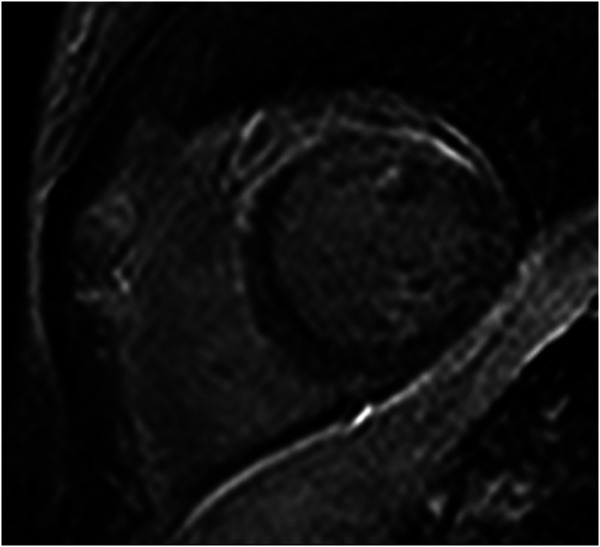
Initial cardiac magnetic resonance imaging demonstrating subepicardial delayed enhancement primarily affecting the anterior myocardium.
